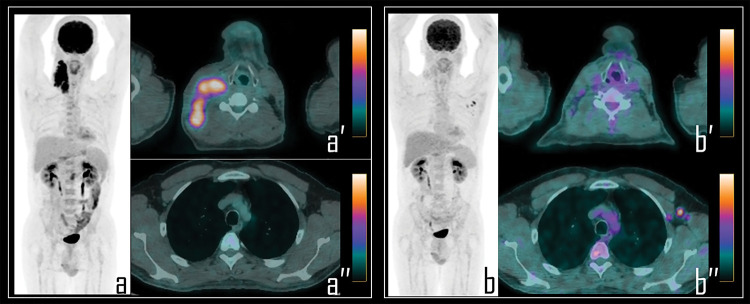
A 25-year-old male patient underwent 2-[^18^F]FDG PET/CT during the staging of *Hodgkin’s lymphoma*. PET (a) demonstrated pathologic tracer uptake in right lateral cervical lymphadenopathies. Axial PET/CT view well displays pathologic lymphadenopathies in the neck (a′) while no foci of uptake were detected in the axillary regions (a″). Subsequently, patient was submitted to chemotherapy and, 9 days before the interim PET/CT, to COVID-19 vaccine administration in the left arm. Note: Interim PET/CT (b) showed response to therapy with reduction of the uptake in the neck, more evident in axial PET/CT view (b″); conversely, three lymph nodes with moderate 2-[^18^F]FDG uptake were detected in the left axilla, as displayed in axial PET/CT view (b″), presenting SUVmax 2.8. These findings were linked to the previous vaccine administration.
